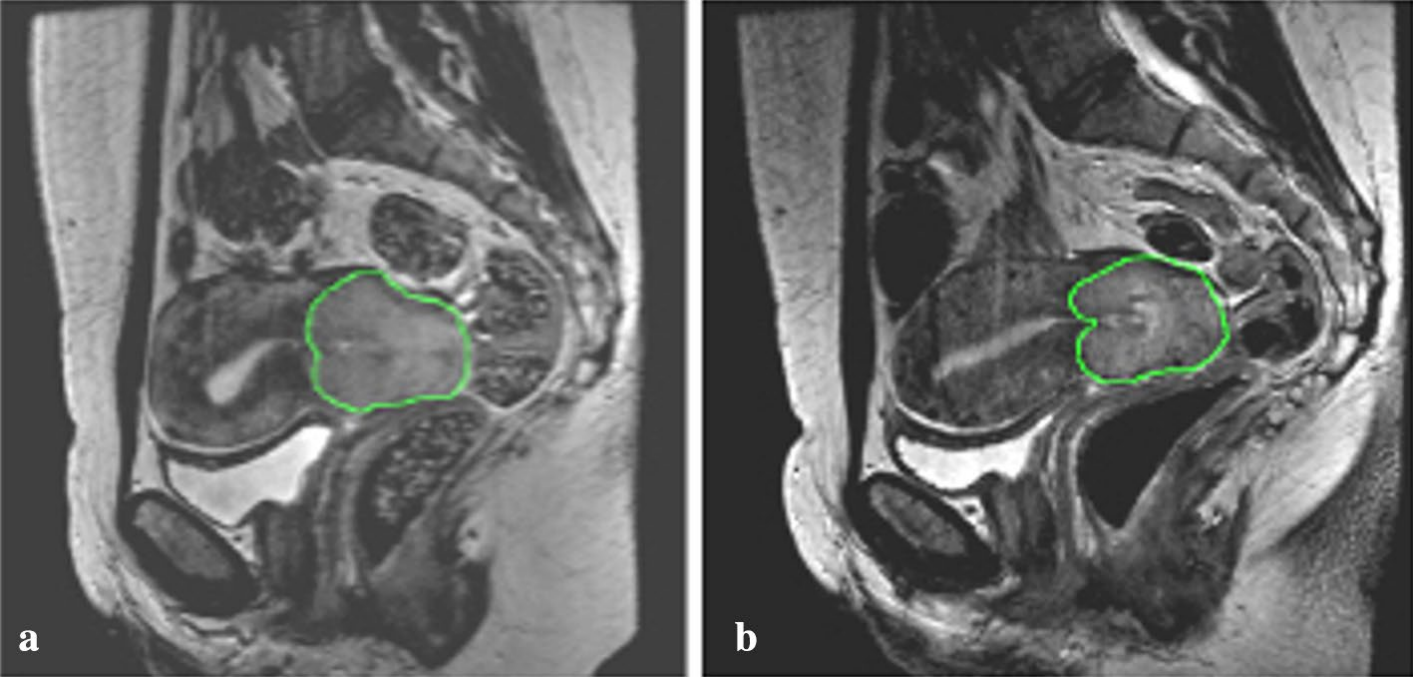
a Baseline GTV delineation on T2W MRI for one of the study participants. b Early treatment response evaluation GTV on T2W MRI, single modality, for the same study participant, showing a minor volume reduction on visual assessment
